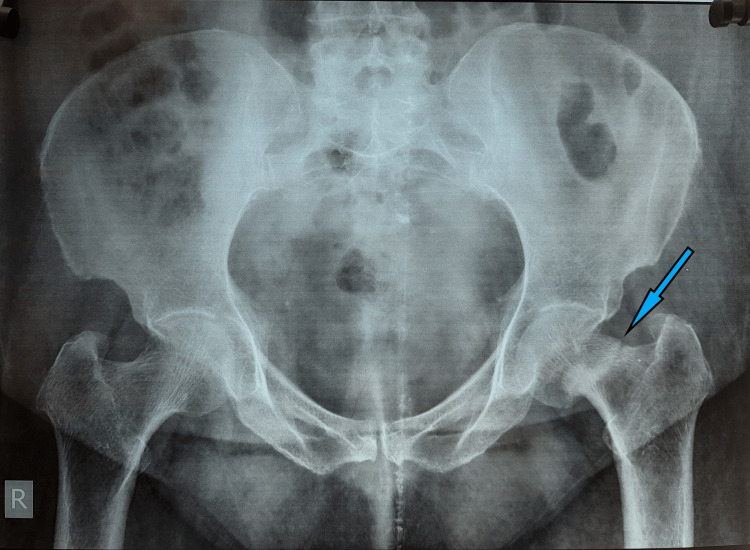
Pelvic X-ray showing a sclerotic line running along the left femur neck, suggestive of a stress femur neck fracture
